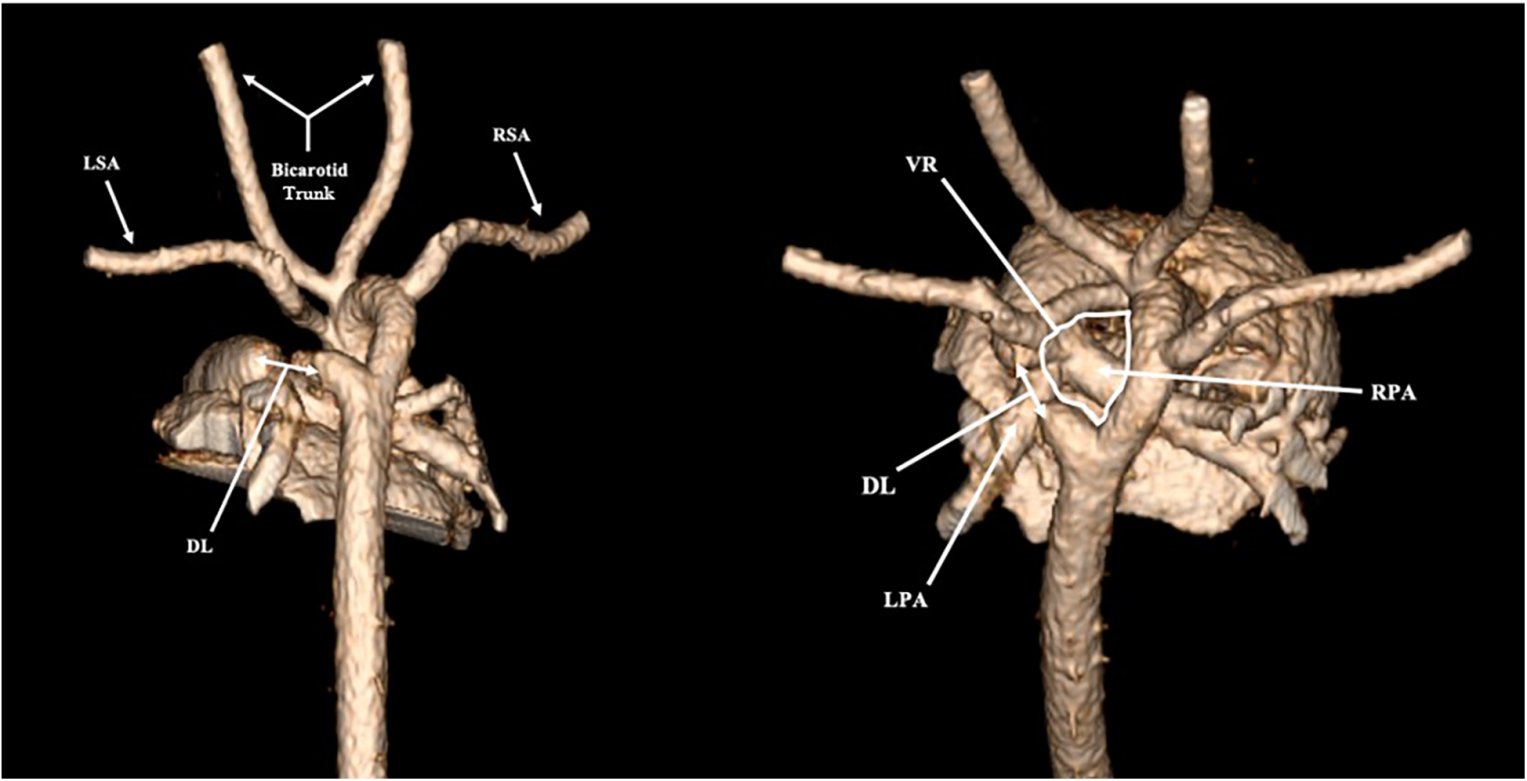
Three-dimensional reconstruction of cardiac computed tomography (CCTA). LSA, left subclavian artery; RSA, right subclavian artery; DL, ductal ligament; VR, vascular ring; LPA, left pulmonary artery; RPA, right pulmonary artery.
